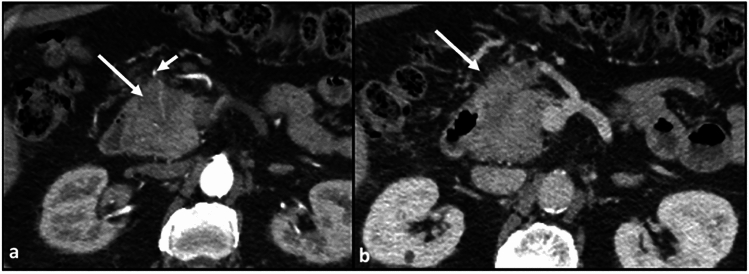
Axial CT sections with contrast in the arterial phase (a) demonstrates heterogenous hypodense mass in the anterior pancreatic head (long arrow), with disease infiltrating adjacent structures and encasing the gastroduodenal artery (short arrow). Axial CT section with contrast in the portal venous phase (b) demonstrates infiltration of disease through the dorsal mesoduodenum into the duodenum, with early infiltration into the transverse mesocolon as well (arrow)
